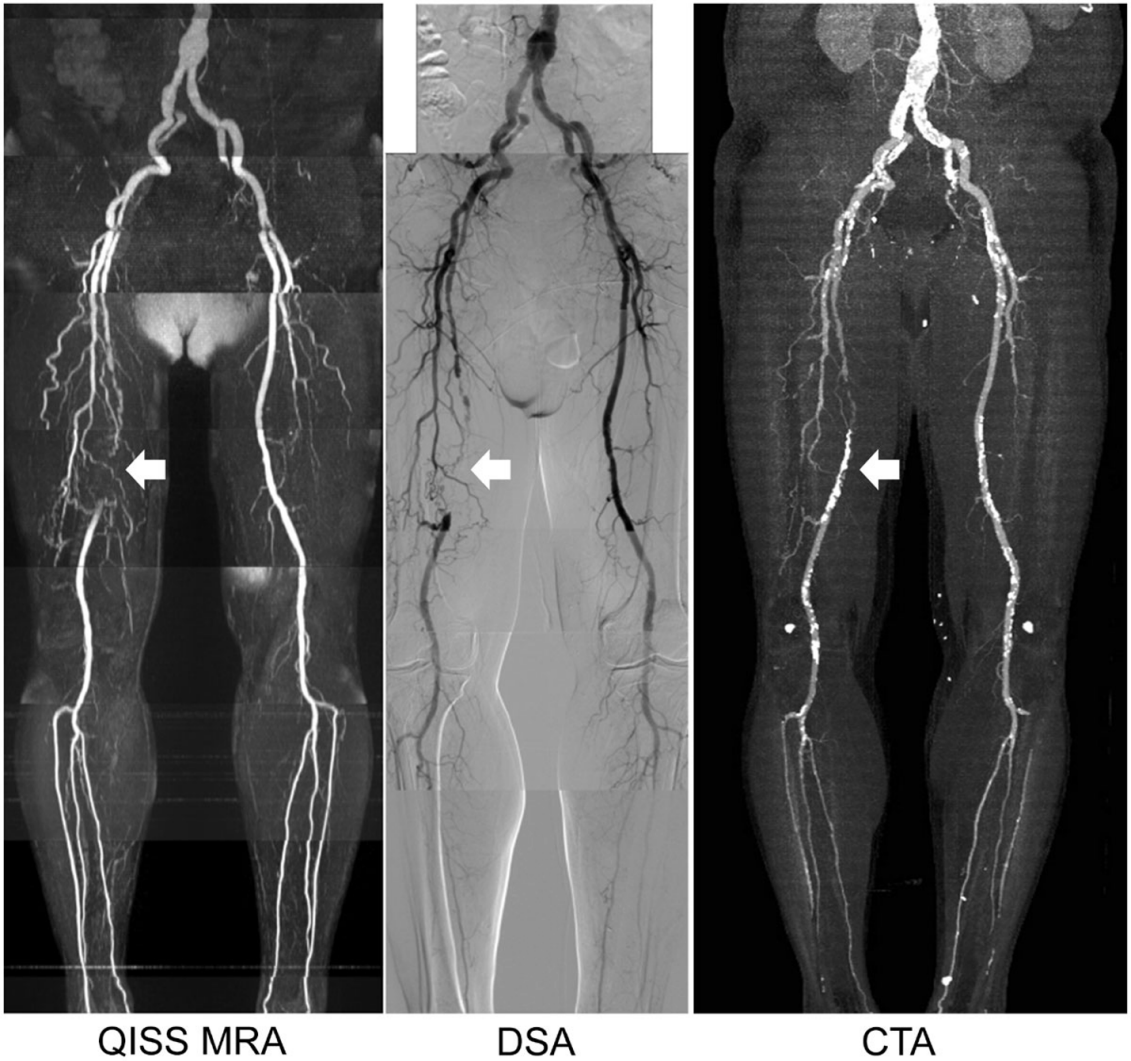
Representative QISS MRA, DSA and CTA images in a 75-year-old man with right femoral superficial artery occlusion (arrows).
